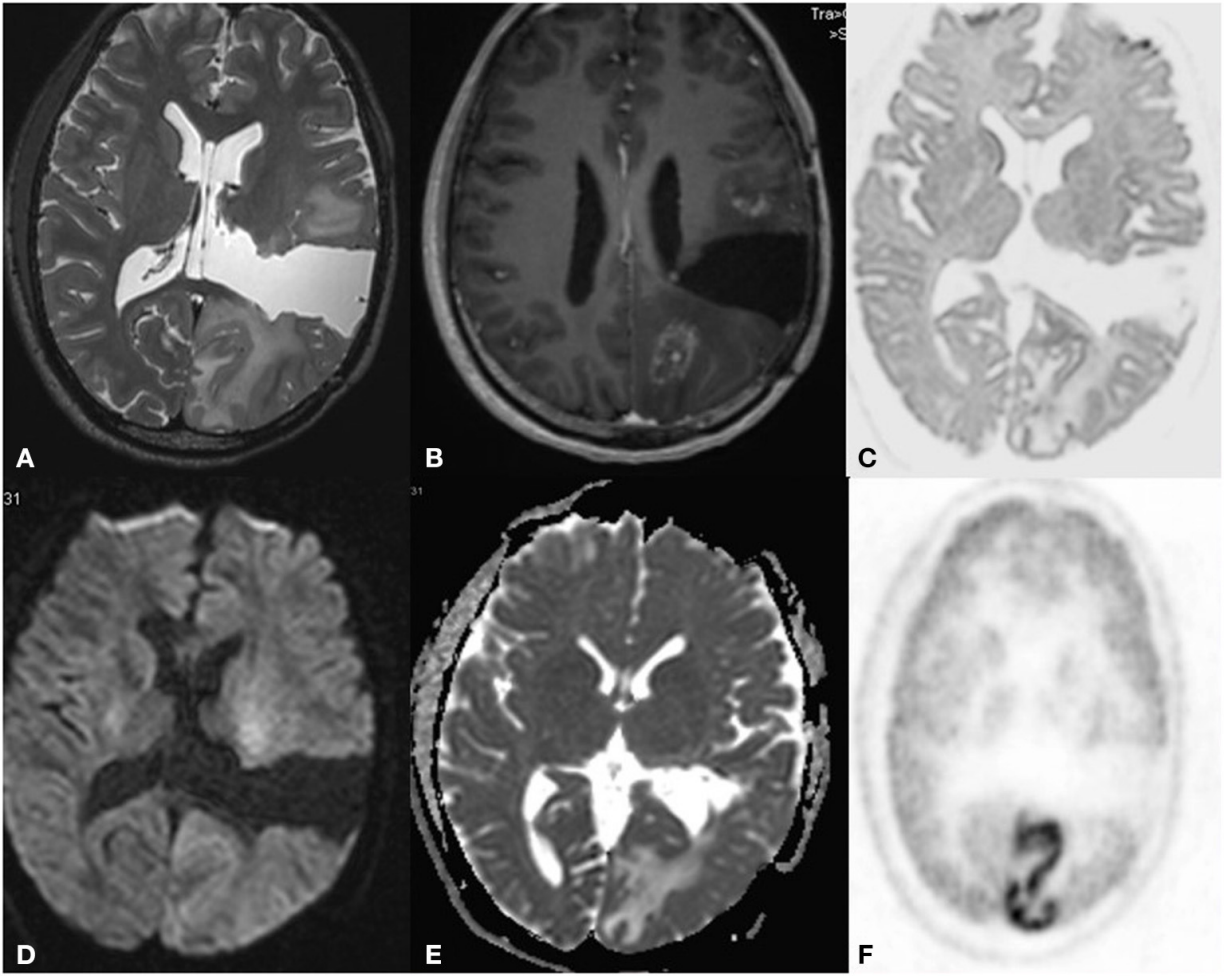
A 32 year old male postoperative, post-radiotherapy case of Anaplastic mixed oligoastrocytoma left thalamus, 3 year follow up MRI FDG PET axial images T2W (A) post-contrast T1 (B) Inverted DWI (C) DWI b2000 (D) ADC (E) NAC PET (F) show a postoperative cavity in the left parietal region. Anterior and posterior to this there is a heterogeneously enhancing lesion with elevated perfusion. The lesion shows DWI restriction with hypermetabolism on PET. This is suggestive of recurrence, but there is a large area of gyral enhancement due to SMART Syndrome. There is a false positive uptake on PET.
